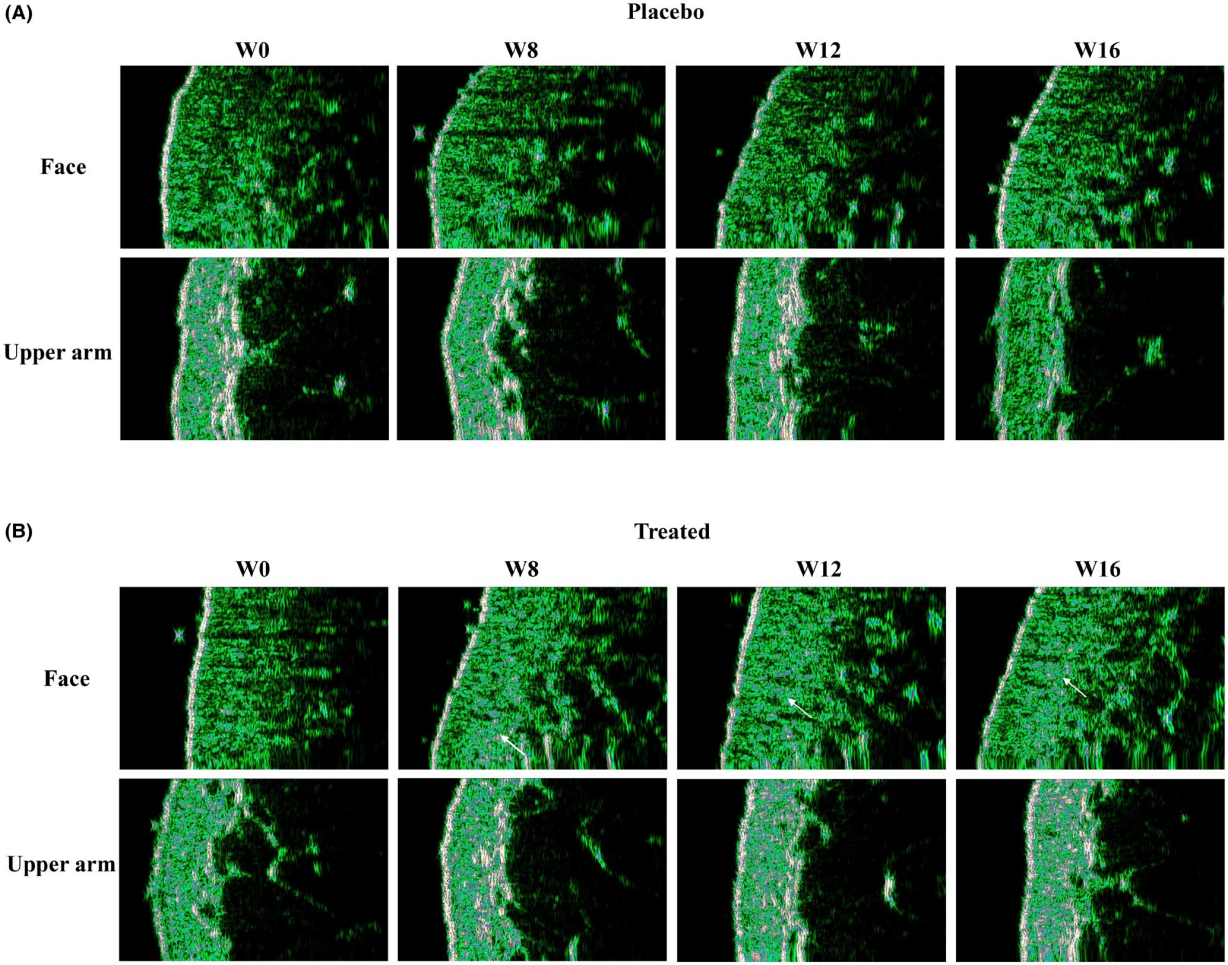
Ultrasound images of the dermis of the face and upper arms: (A) placebo group; (B) treated group.
